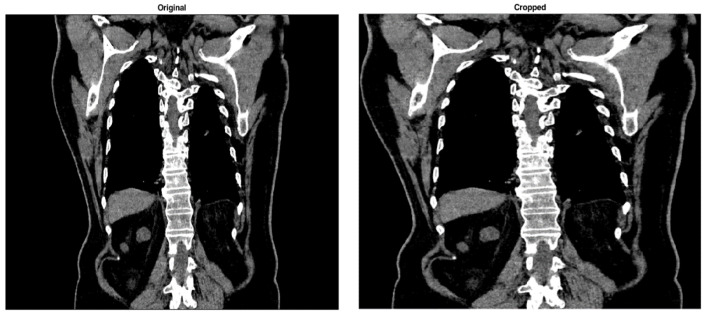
A CT image before (on the left) and after removing its black margin (on the right).
